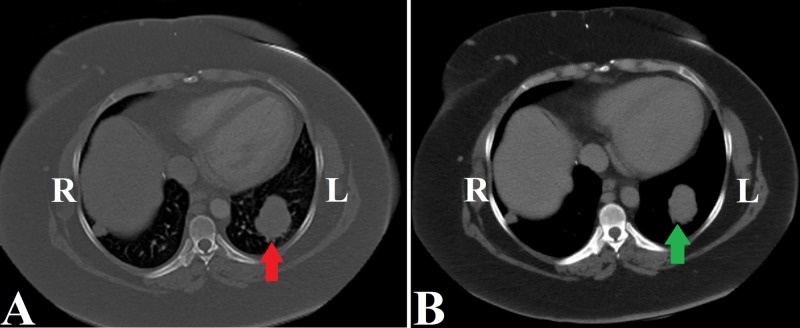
Chest CT showing: (A) multiple pulmonary nodules with the largest nodule measuring 4.7 cm x 3.8 cm (red arrow); (B) reduced size of the largest nodule to 4.1 cm x 3.1 cm upon follow-up (green arrow). R, right; L, left
